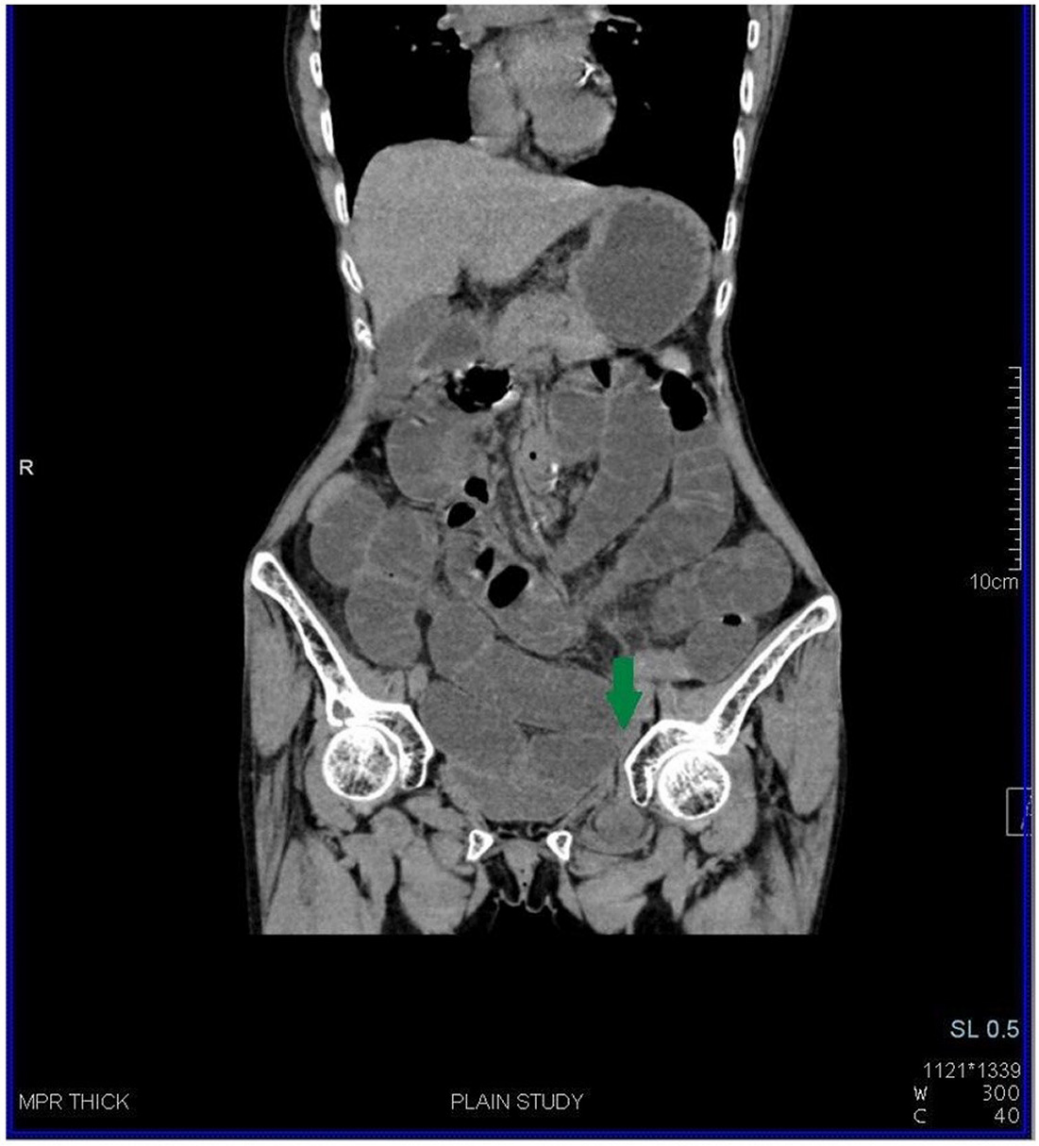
This is a plane coronal computed tomography showing left obturator hernia and fluid filled dilated proximal small bowel loops with maximum luminal diameter of 3.6 cm with collapsed terminal ileum and large bowel loops.
